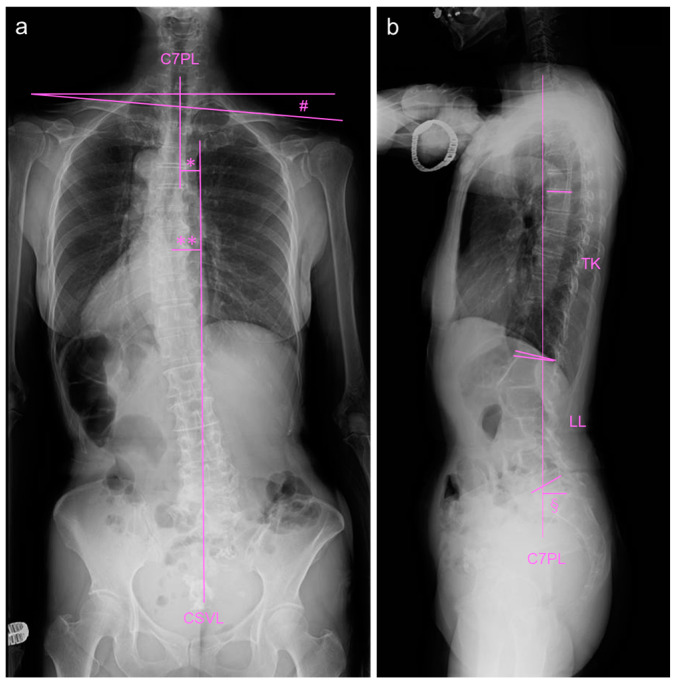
Standing whole-spine radiographs at baseline. (a) Antero-posterior whole-spine radiograph. # indicates clavicle angle, * indicates C7-CSVL measurement, and  indicates apex-CSVL measurement. (b) Lateral whole-spine radiograph. C7PL: C7 plum line, TK: thoracic kyphosis, LL: lumbar lordosis, sagittal vertical axis: SVA. § indicates sagittal vertical axis (SVA).
